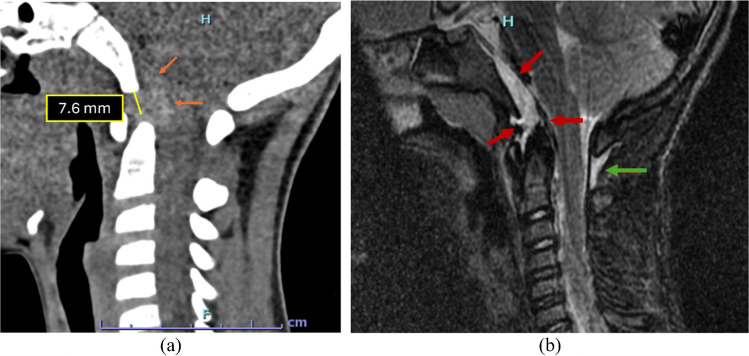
An 8-year-old child following a motor vehicle collision, presenting with neurological deterioration, neck pain, and stiffness. (a) Sagittal CT demonstrates an increased basion–dens interval. Given the patient’s age (<10 years), the basion–cartilaginous dens interval (BCDI) cut-off was applied (*N*<7.2 mm) with a retroclival hyperdensity (*orange arrows*) noted, raising suspicion for a distraction-type injury. (b) Subsequent MRI shows fluid within the basion–dens interval and stripping of the tectorial membrane from the clivus with associated hemorrhage (*red arrows*). There is also fluid seen in the C1-2 interspinous space (*green arrow*). The patient was managed with immobilization and exhibited gradual clinical recovery
